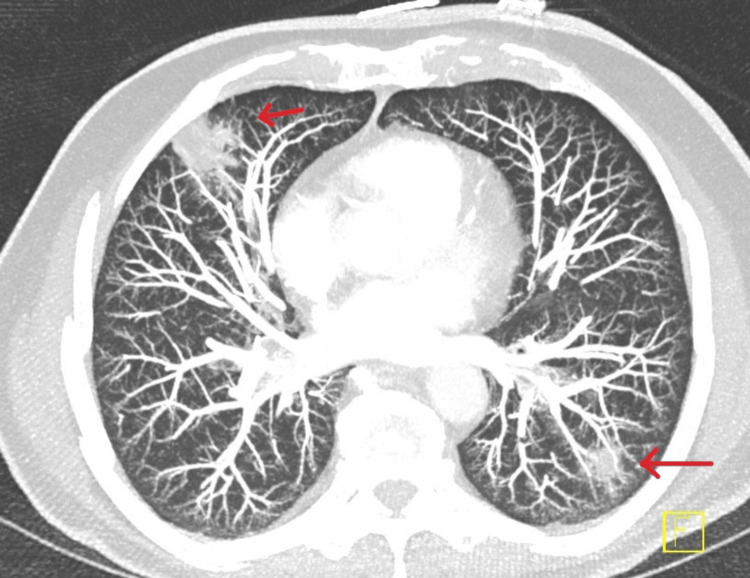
Contrast-enhanced computed tomography (CT) of the chest demonstrating multifocal bilateral peripheral nodular and mass-like opacities Contrast-enhanced CT of the chest demonstrating multifocal bilateral peripheral nodular opacities (red arrows), consistent with septic pulmonary emboli in the setting of right-sided infective endocarditis.
